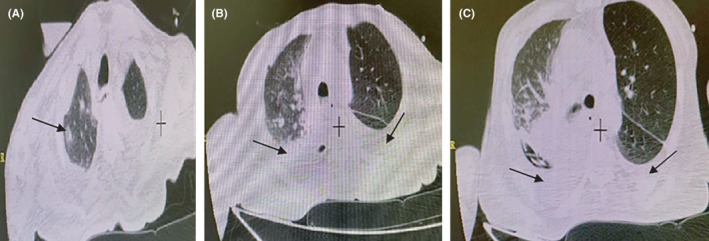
HRCT chest imaging. On the fourth day of hospitalization, and after implanting a chest tube. A, Patchy consolidation at the apex and centrilobular lung nodules on the right lung, B, moderate volume of pleural effusion in the left lung, and C, mildly volume hydropneumothorax with lower lung consolidation collapse on the right side after chest tube implantation
